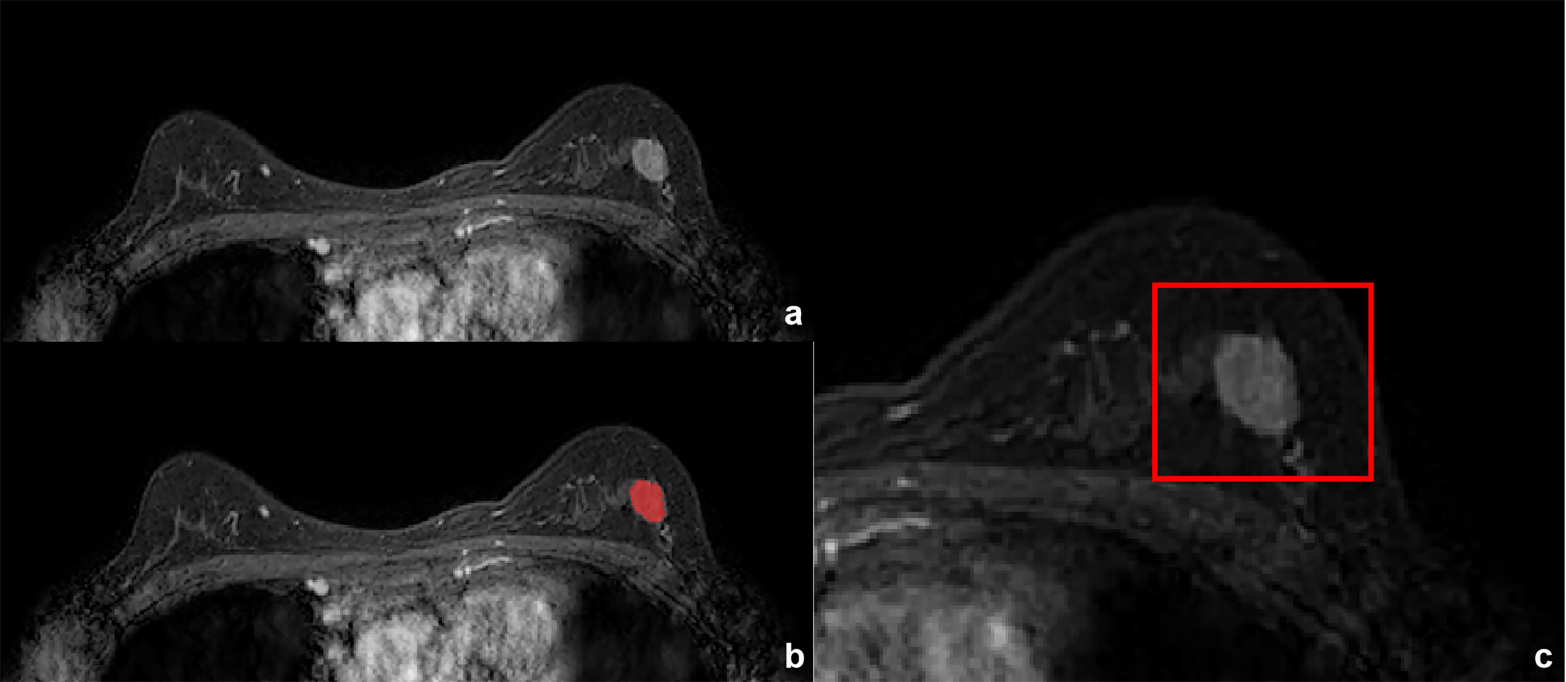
Invasive breast cancer in a 57-year-old woman. (A) T1-weighted postcontract image. (B) Annotation masks (in red) are superimposed over the MR images. The annotations were made by radiologists using ITK-snap. (C) The anchor box was generated by the Faster R-CNN model after the segmentation.
